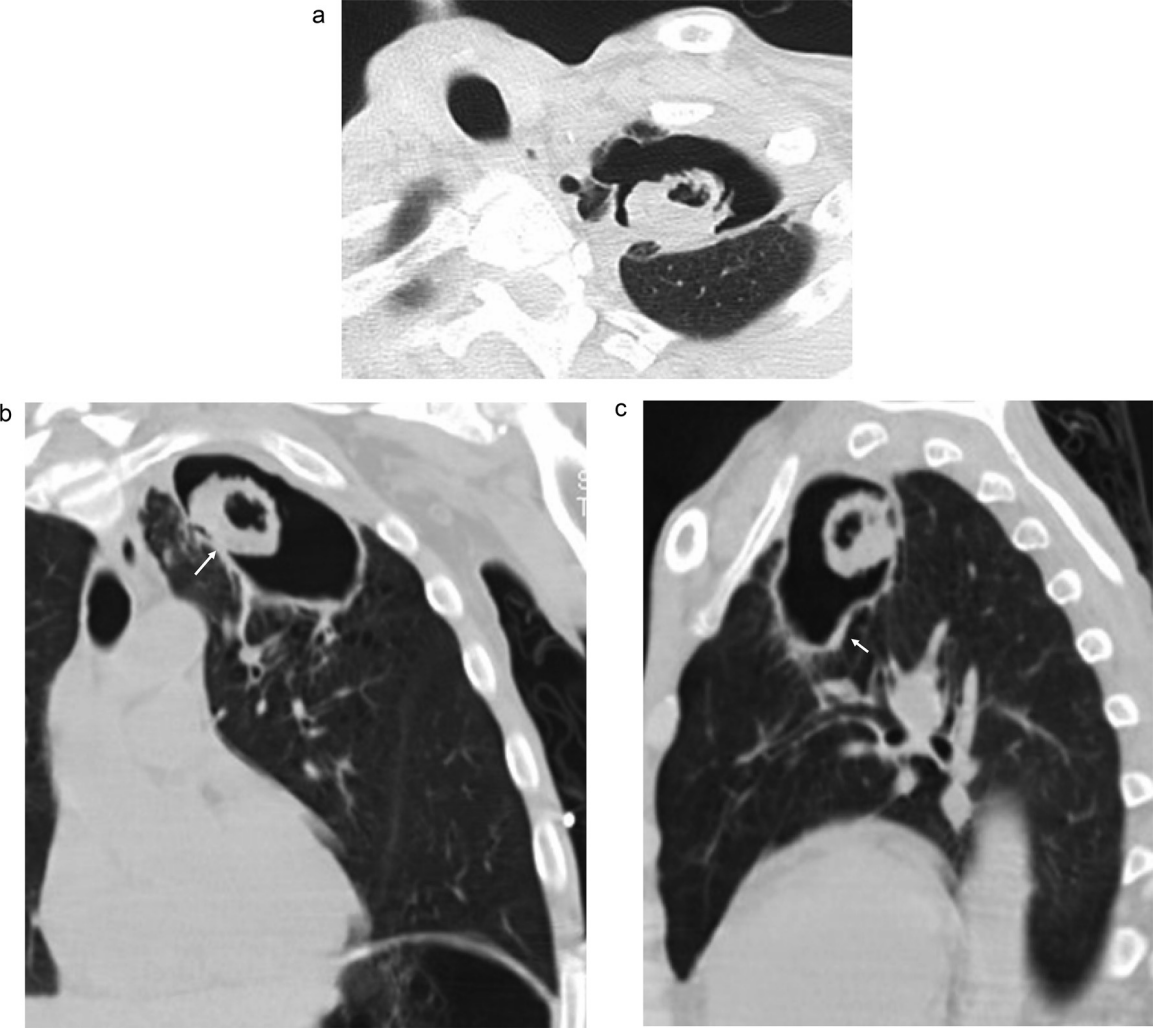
(a,b,c) Axial, coronal and sagittal CT scans of the chest show the intra-cavitary mass with surrounding ‘air-crescent’ (Fig. 2b arrow). The wall of the cavity is thickened (Fig. 2c arrow).
